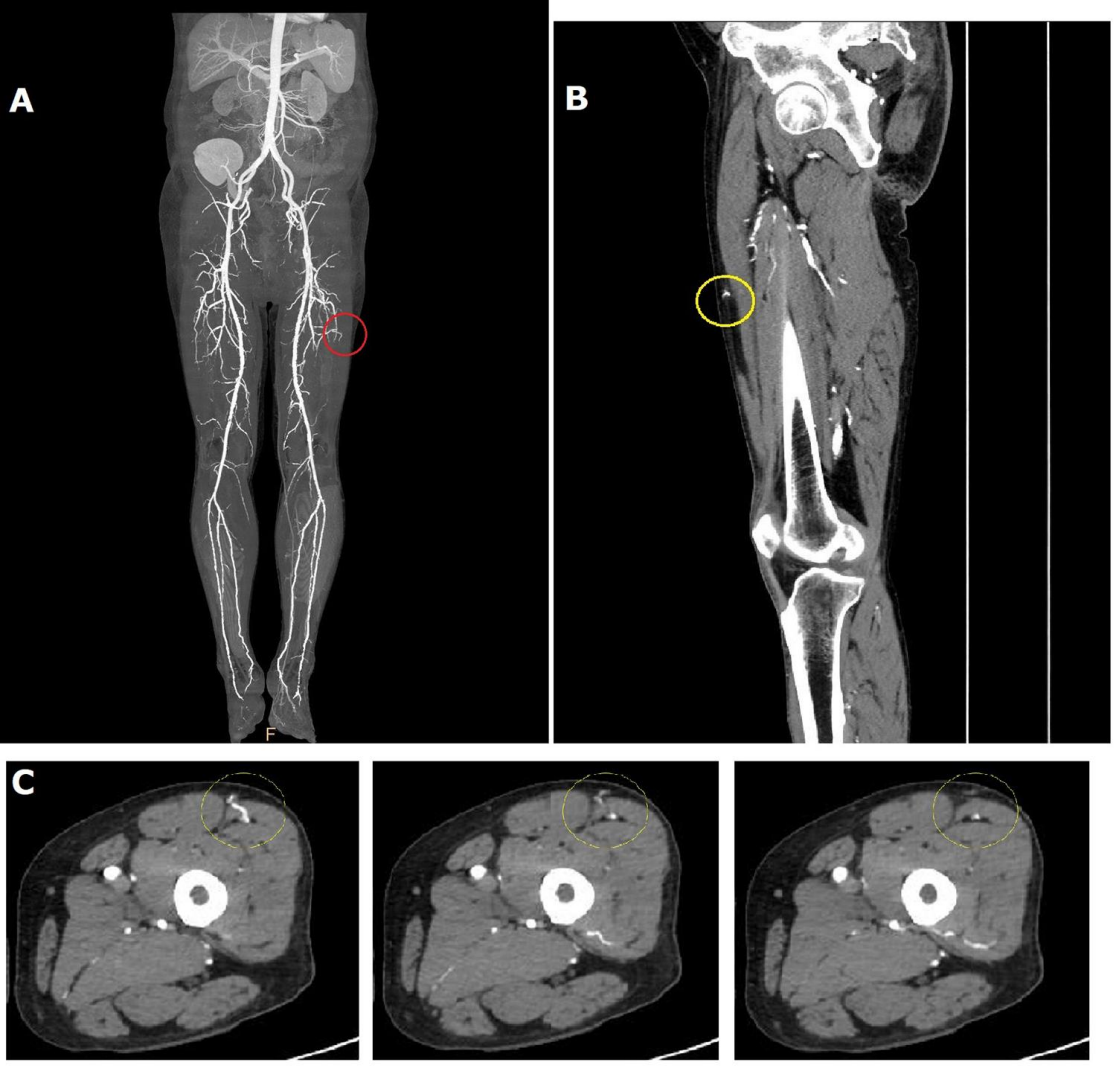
Preoperative computed tomographic angiography (CTA) findings. Contrast timing was set to start scanning when the iliac artery displayed intense enhancement, generally between 35 and 40 s. Though severely atherosclerotic with diffuse calcific plaques, grossly intact major vasculature patency was confirmed in the left lower limb donor and recipient sites (A) The flap was elevated based on a perforator with a robust audible Doppler signal in the customary circular area for anterolateral thigh flap dissection which corresponded to a musculocutaneous perforator visible on CTA [(B), sagittal view; (C), axial view], indicated by yellow circles. The area of the flap perforator is also depicted in a red circle in A.
